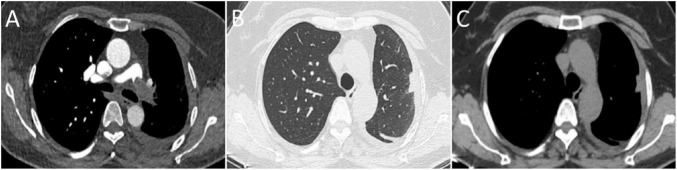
Computed tomography (CT) findings. (A) Computed tomography pulmonary angiography (CTPA) showing a filling defect in the left main pulmonary artery. (B,C) Non-contrast chest CT shows interstitial thickening at the peripheral region of the left upper lobe and focal nodular thickening of the left pleura.
